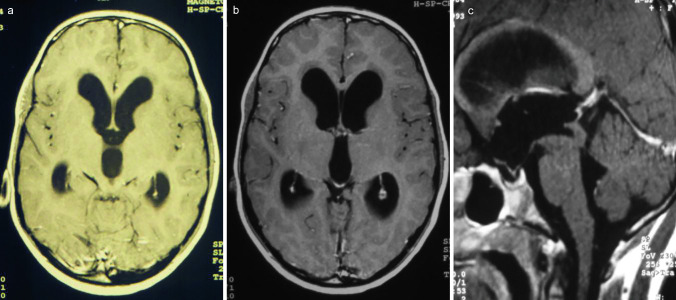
Preoperative axial a, b, and sagittal c MR images showing tri-ventricular occlusive hydrocephalus due to compression of the aqueduct by a tectal lesion, suspected for low-grade tumor
